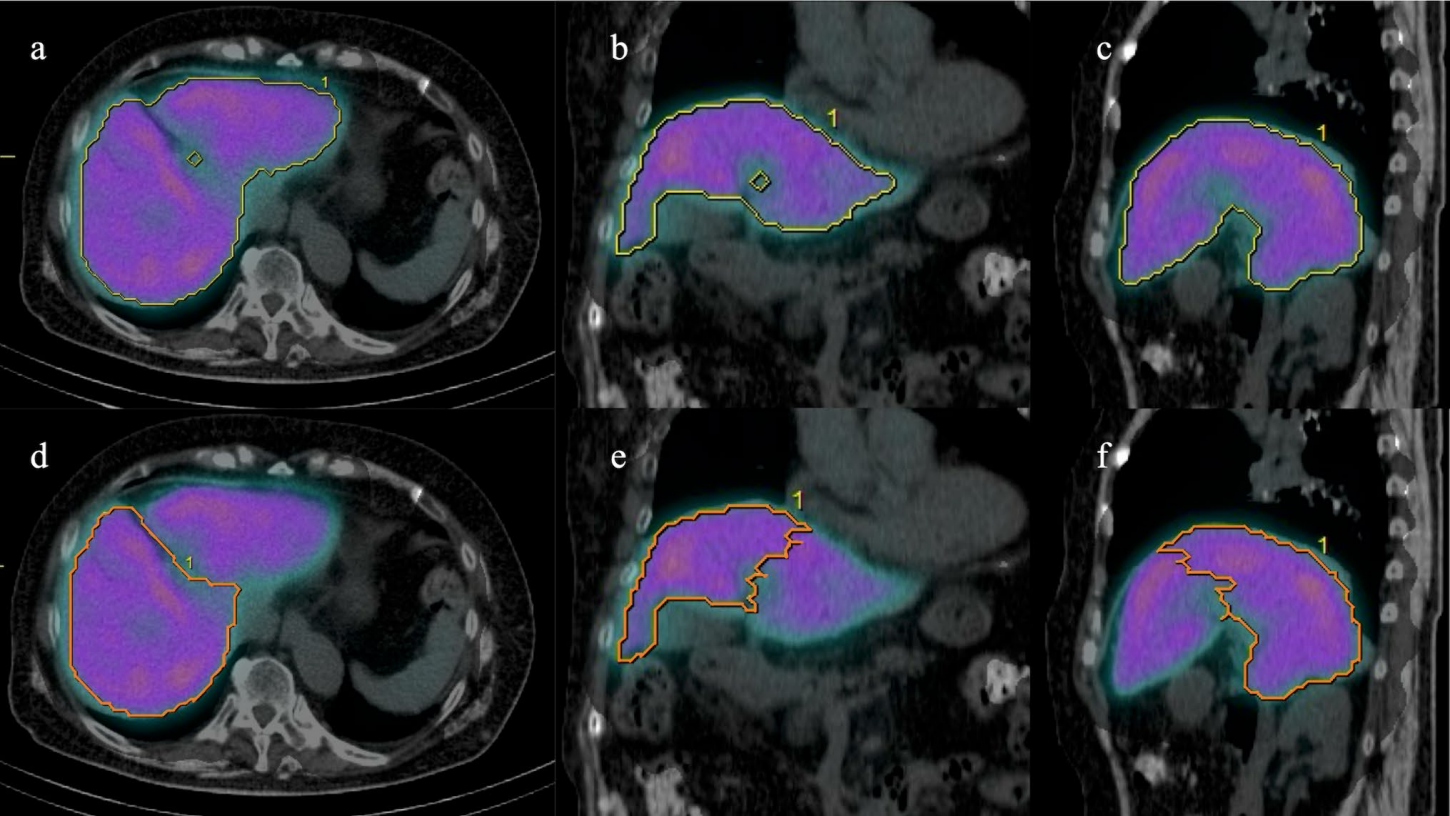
Calculation of functional liver volume of total liver (t-FLV) and functional liver volume of remnant liver (r-FLV) for left lateral sectionectomy. a (Transaxial image), b (coronal image), and c (sagittal image) show calculation of t-FLV (yellow line). The software (RAVAT) automatically contoured whole liver above standardized uptake value (SUV) ≥ 20. d (Transaxial image), e (coronal image), and f (sagittal image) show calculation of r-FLV (orange line). The resection line was drawn manually
